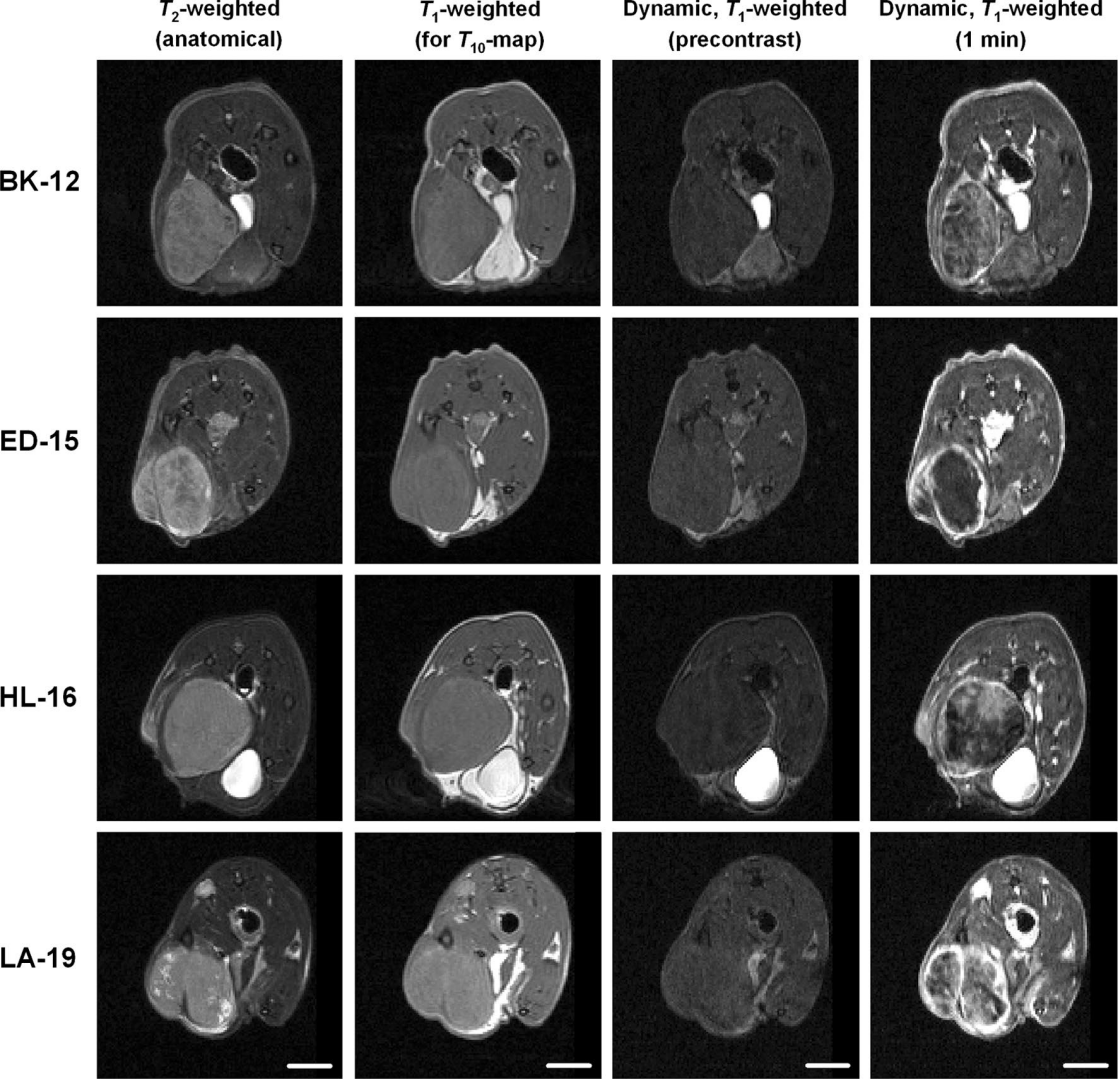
MR images of a representative BK-12, ED-15, HL-16, and LA-19 tumor. Displayed from left to right are anatomical *T*
